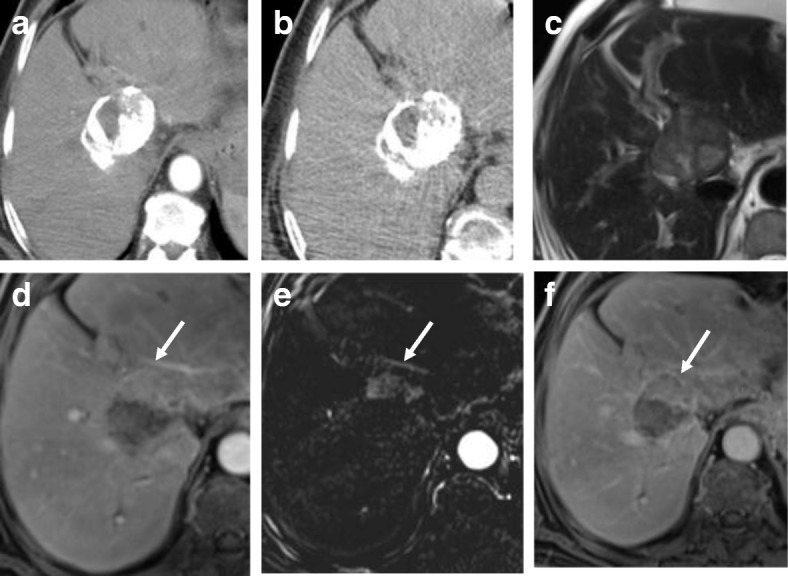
CT versus MRI after TACE. a Arterial phase CT shows heterogenous lipiodol condensation without appreciable enhancement. b Delayed phase CT shows heterogenous lipiodol condensation without appreciable washout. c T2-WI shows a heterogenous signal. d Enhanced T1-WI in the arterial phase shows non-enhancement in most of the lesion with faintly enhancing peripheral nodule (arrow). e Subtracted image confirms non-enhancement in most of the lesion with a faintly enhancing peripheral nodule (arrow). f Enhanced T1-WI in the delayed phase shows washout of the faintly enhancing peripheral nodule (arrow). Final diagnosis is LR-TR viable which is highlighted by MRI, not by CT
